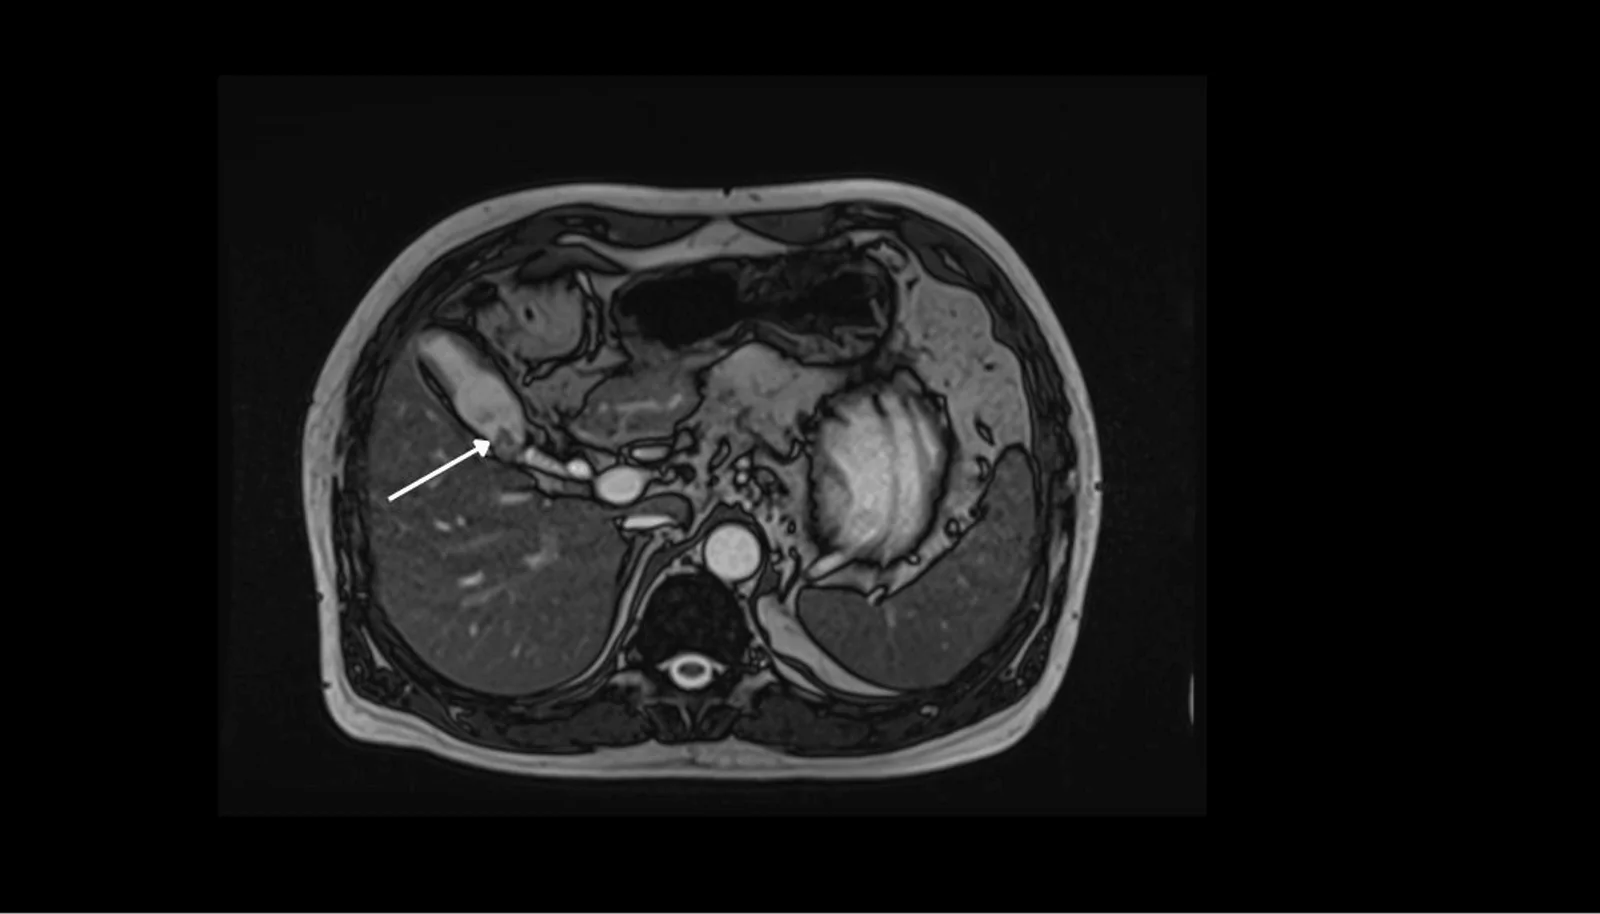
Magnetic resonance cholangiopancreatography (MRCP) T2-weighted axial image showing filling defects within the neck of the gallbladder measuring approximately 10 mm The arrowhead points toward the gallbladder neck.
